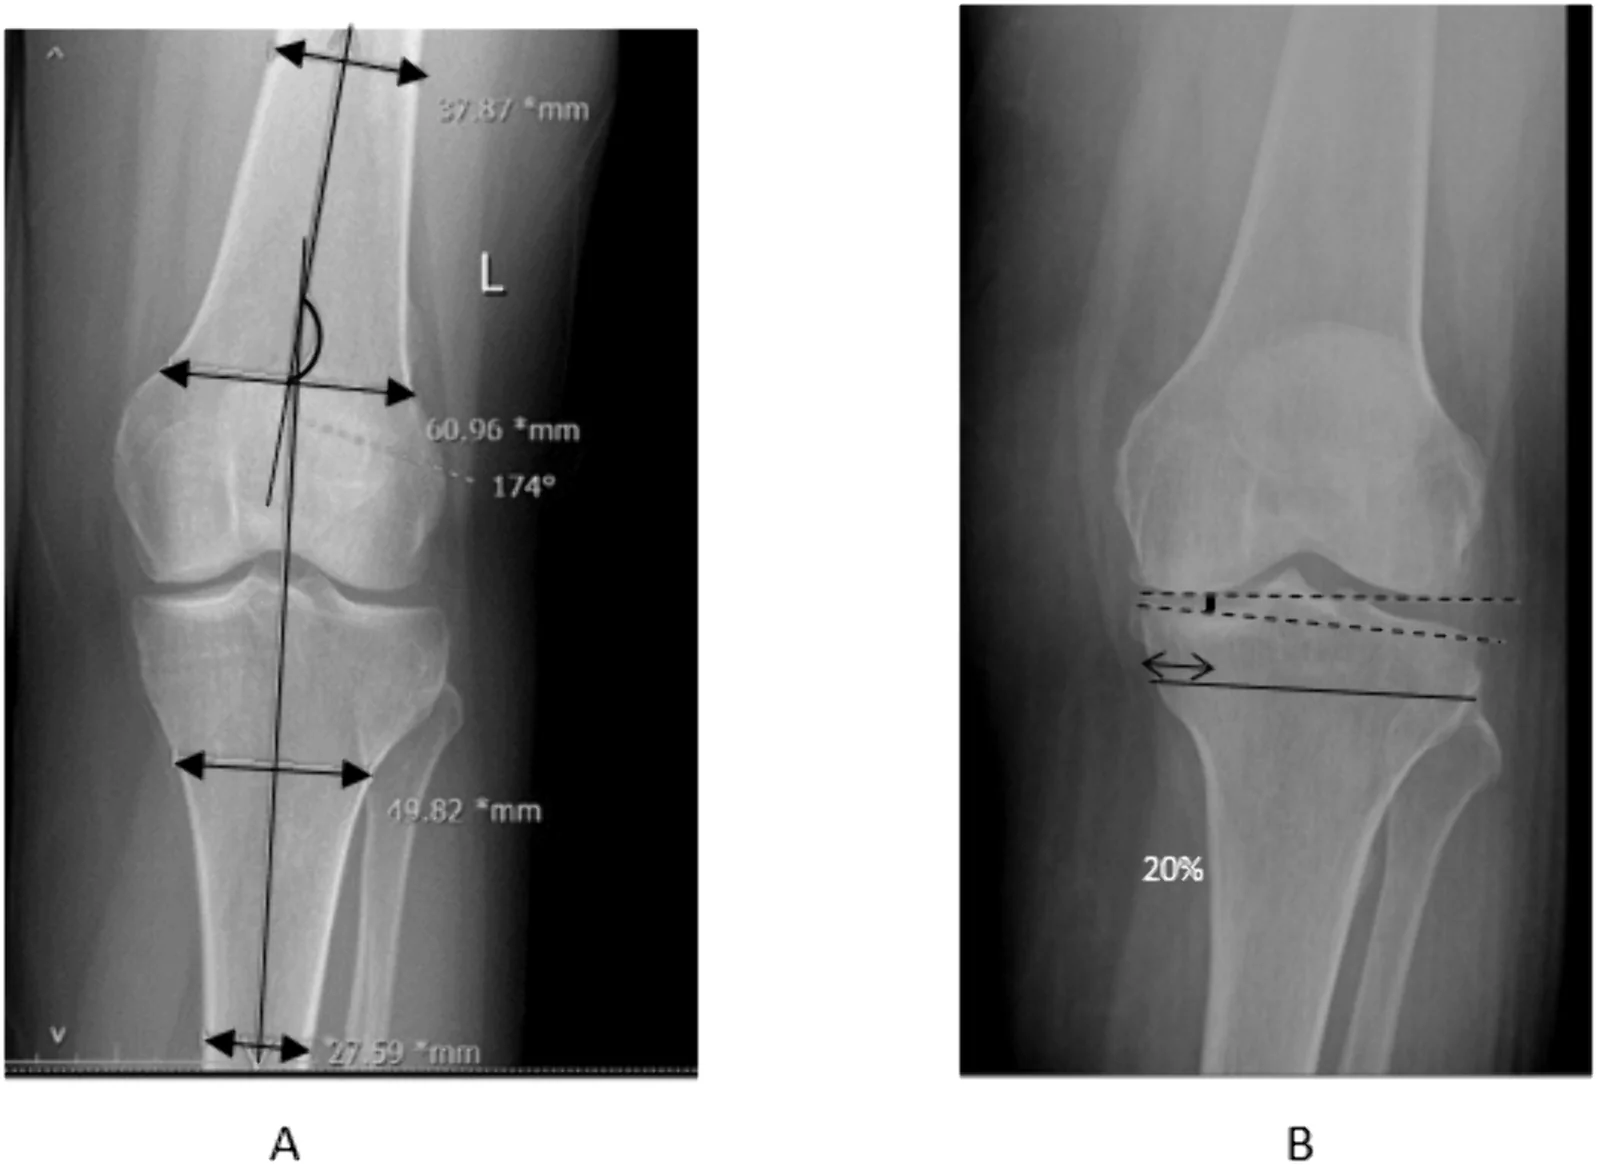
Radiographic Knee Metrics. A. Measurement of the femorotibial angle (FTA): The double arrows indicate the proximal and distal widths of the femoral and tibial shafts, respectively. Midpoints of these widths were connected to define the anatomical axes of each bone. The angle formed at the intersection of these axes represents the FTA. B. Measurement of the medial joint space width (MJSW): Dashed lines represent tangents to the femoral condyles and tibial plateaus. The solid horizontal line indicates the total width of the proximal tibia and was used to identify a point located 20% from the medial edge (marked by a double arrow). The MJSW was measured at this point as the perpendicular distance between the dashed lines, shown as a solid vertical line marked by an arrow.
